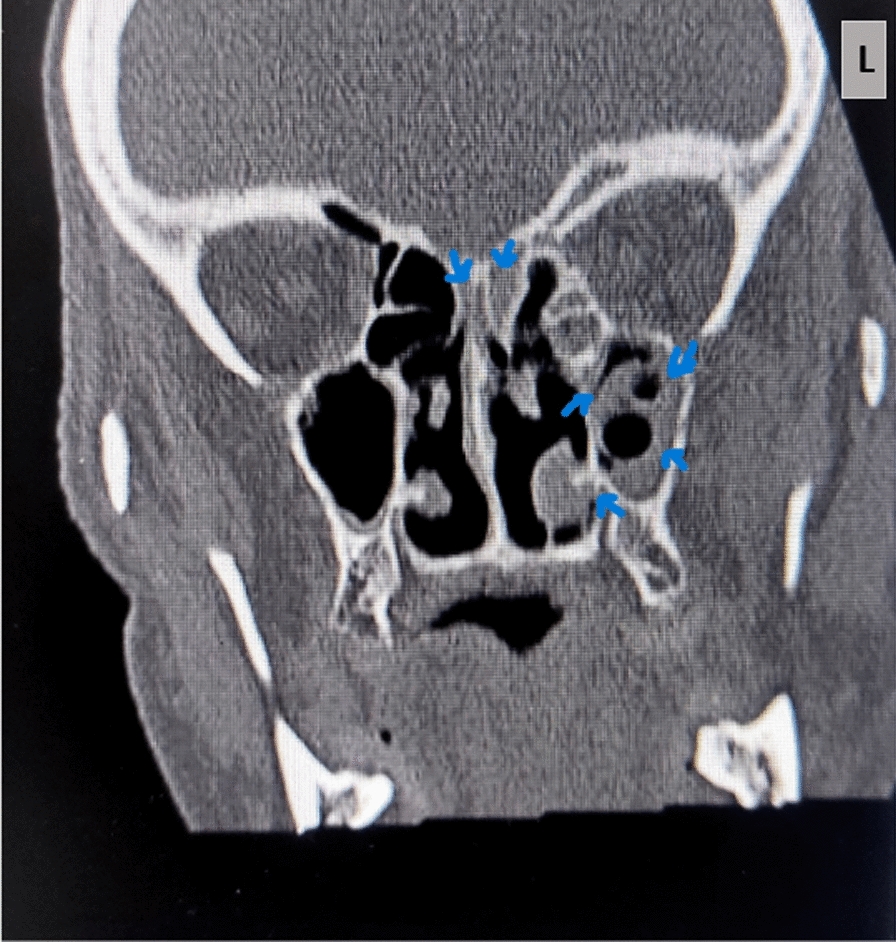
Paranasal sinus computed tomography scan, turbidity, and increased mucus thickness are observed in the maxillary, sphenoid, and frontal sinuses of the right and left ethmoid (arrowhead)
